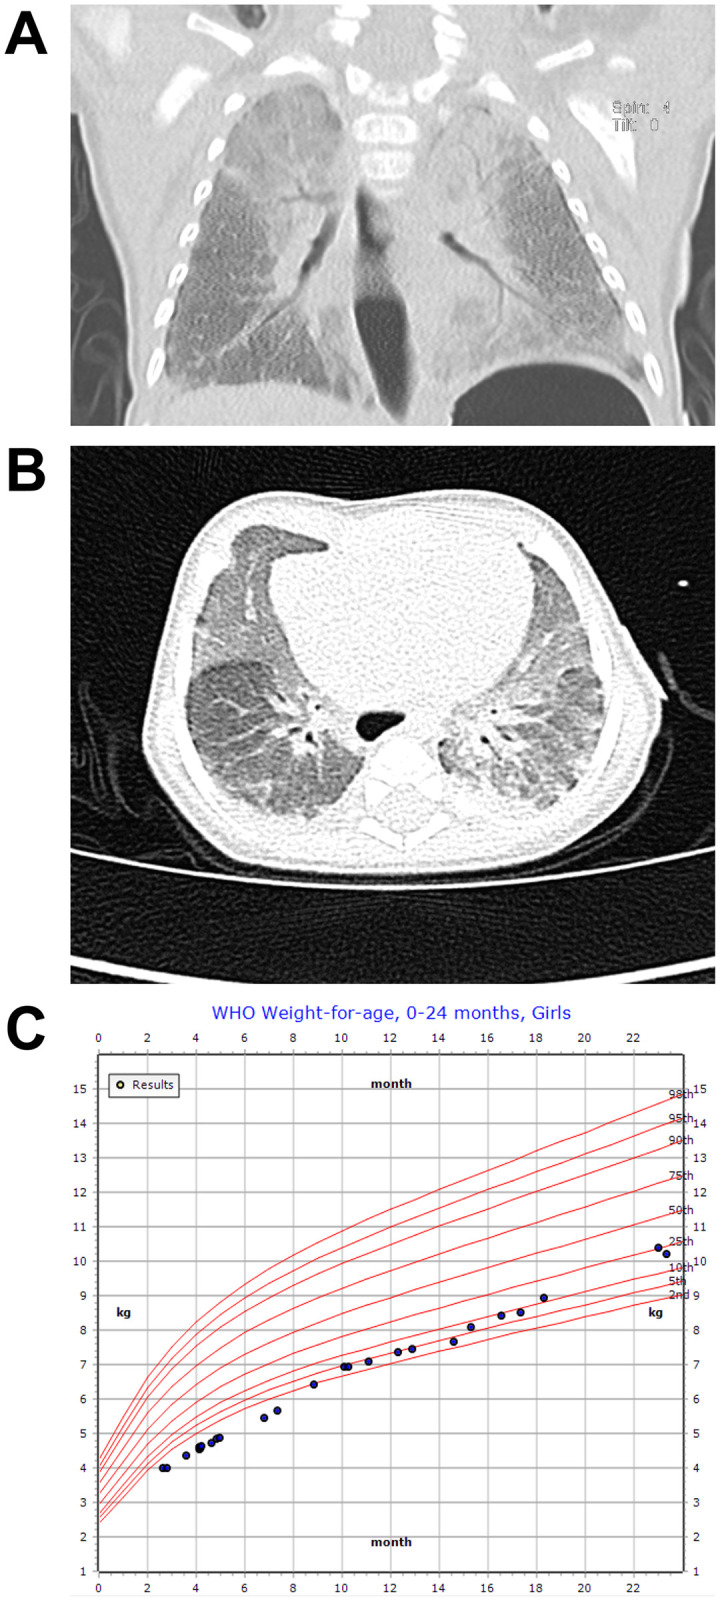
Clinical findings of the proband with homozygous *ARHGAP42* stop-gain variant. (A) Chest X-ray at age 3 months showed bilateral diffuse opacities and low lung volumes; (B) Initial chest CT scan at age 3 months displayed diffuse ground glass opacities with subpleural sparing and patchy subpleural opacities; (C) Patient growth chart for weight through two years of age.
